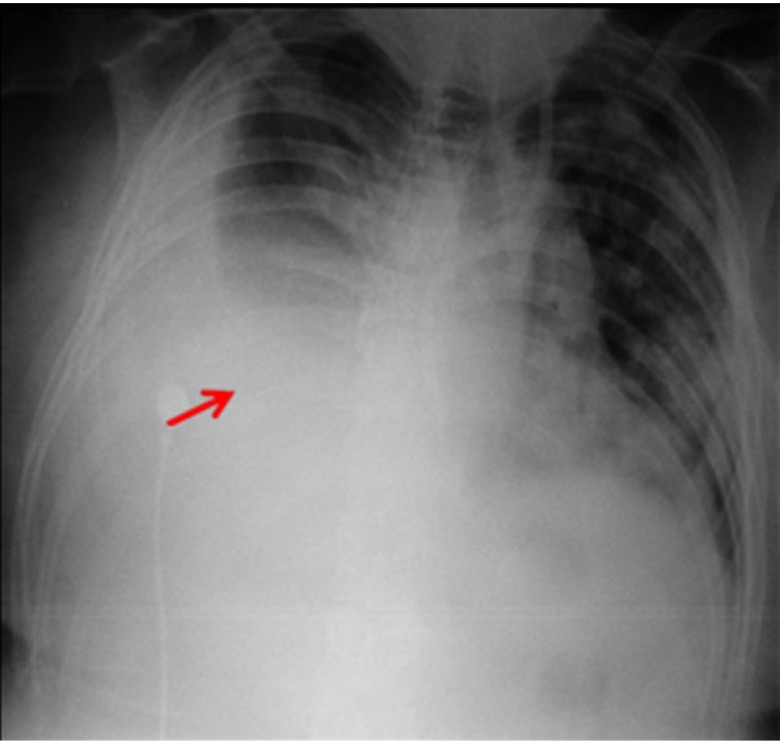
Antero-posterior chest x-ray on admission showing right-sided pleural effusion.
